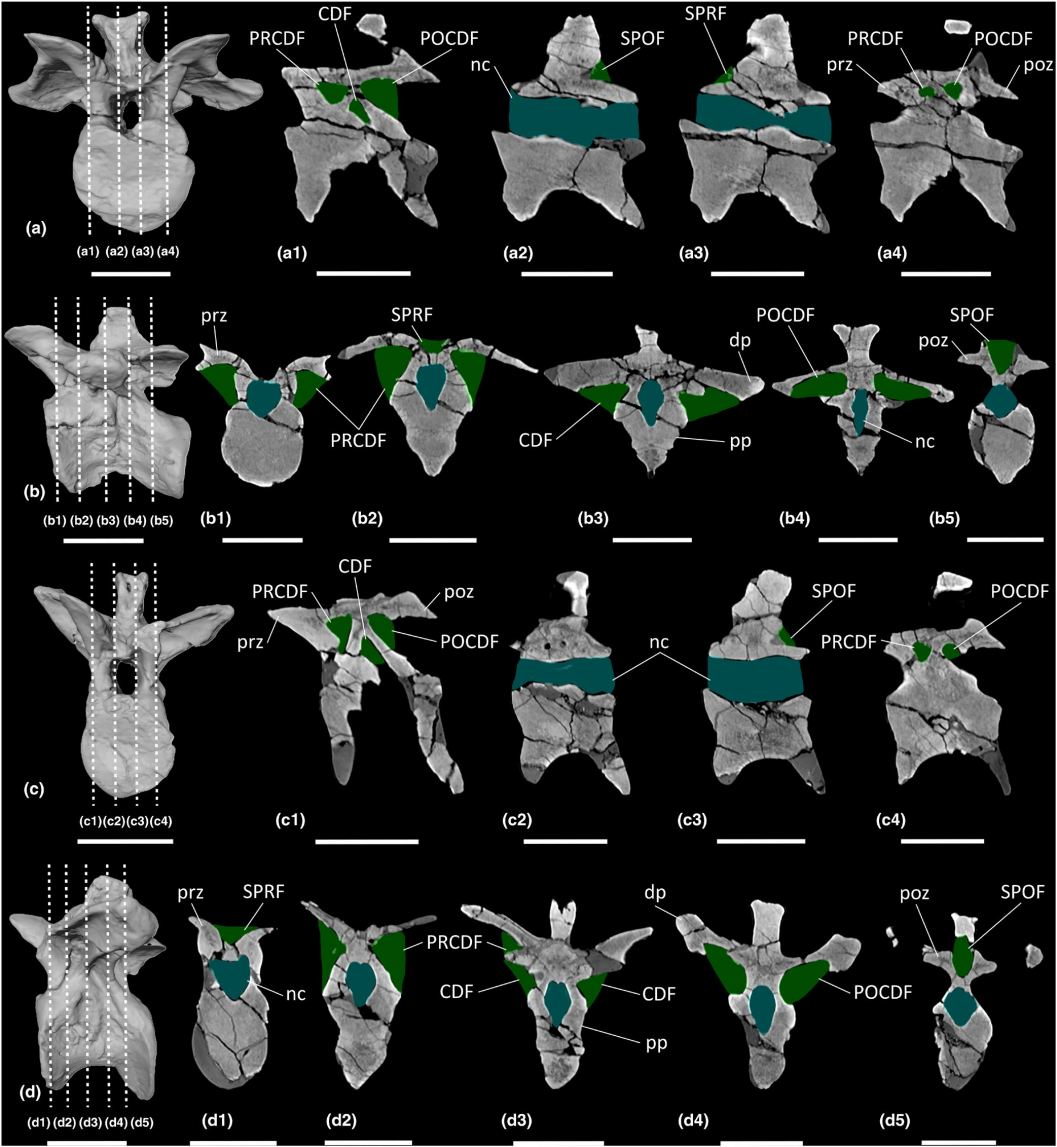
CT scan data of *Plateosaurus longiceps* MB.R.4404 ‘Skeleton 25’ anterior dorsal vertebrae. (a, b) dorsal vertebra 1 MB.R.4404.25, (c, d) dorsal vertebra 3 MB.R.4404.27; (a, c) surface scan anterior view, (b, d) surface scan lateral view; (a1–a4, c1–c4) CT scan slices in parasagittal cross‐section, (b1–b5, d1–d5) CT scan slices in transverse cross‐section. CDF, centrodiapophyseal fossa; dp, diapophysis; nc, neural canal; POCDF, postzygapophyseal centrodiapophyseal fossa; poz, postzygapophysis; pp, parapophysis; PRCDF, prezygapophyseal centrodiapophyseal fossa; prz, prezygapophysis; SPOF, spinopostzygapophyseal fossa; SPRF, spinoprezygapophyseal fossa. Key: Blue highlight, neural canal; green highlight, fossa. Scale bars = 5 cm.
